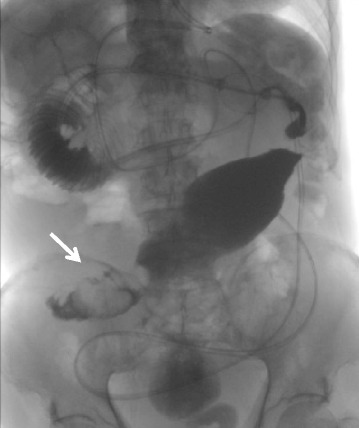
A Gastrografin contrast radiography film revealing a filling defect. Approximately 40 mm in diameter, in the ileum (arrow).
